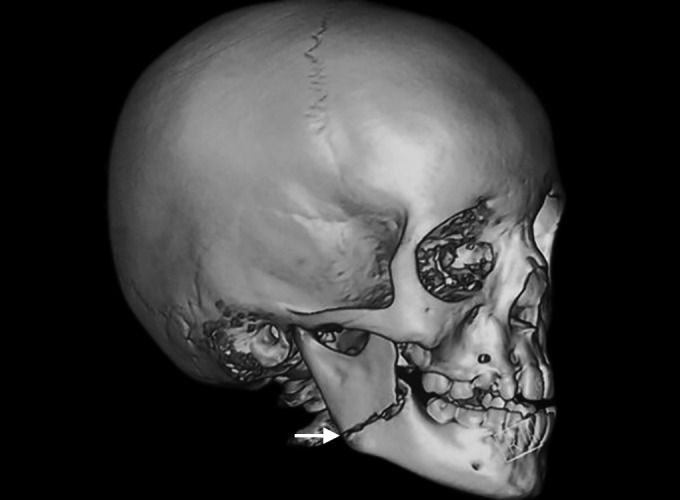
Three dimensional facial CT (arrowhead) with horizontally favorable fracture in relation to right angle of mandible
